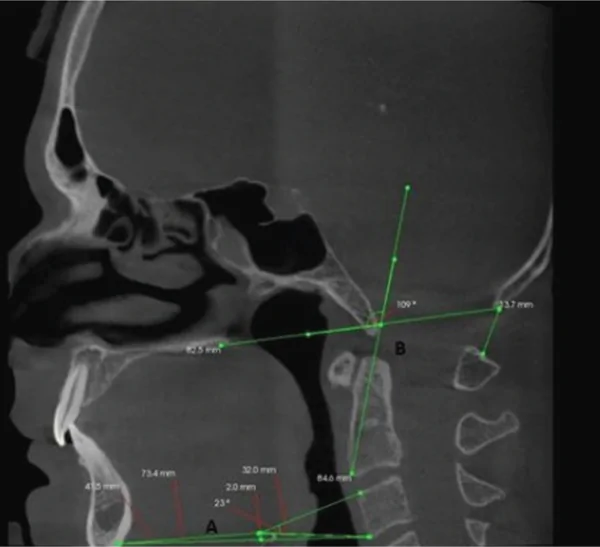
Case 1 pretreatment CBCT reveals a hypodivergent hyoid triangle. (A) The hypodivergent triangle with an angulation of 23 degrees. (B) Cervical vertebrae alignment.
